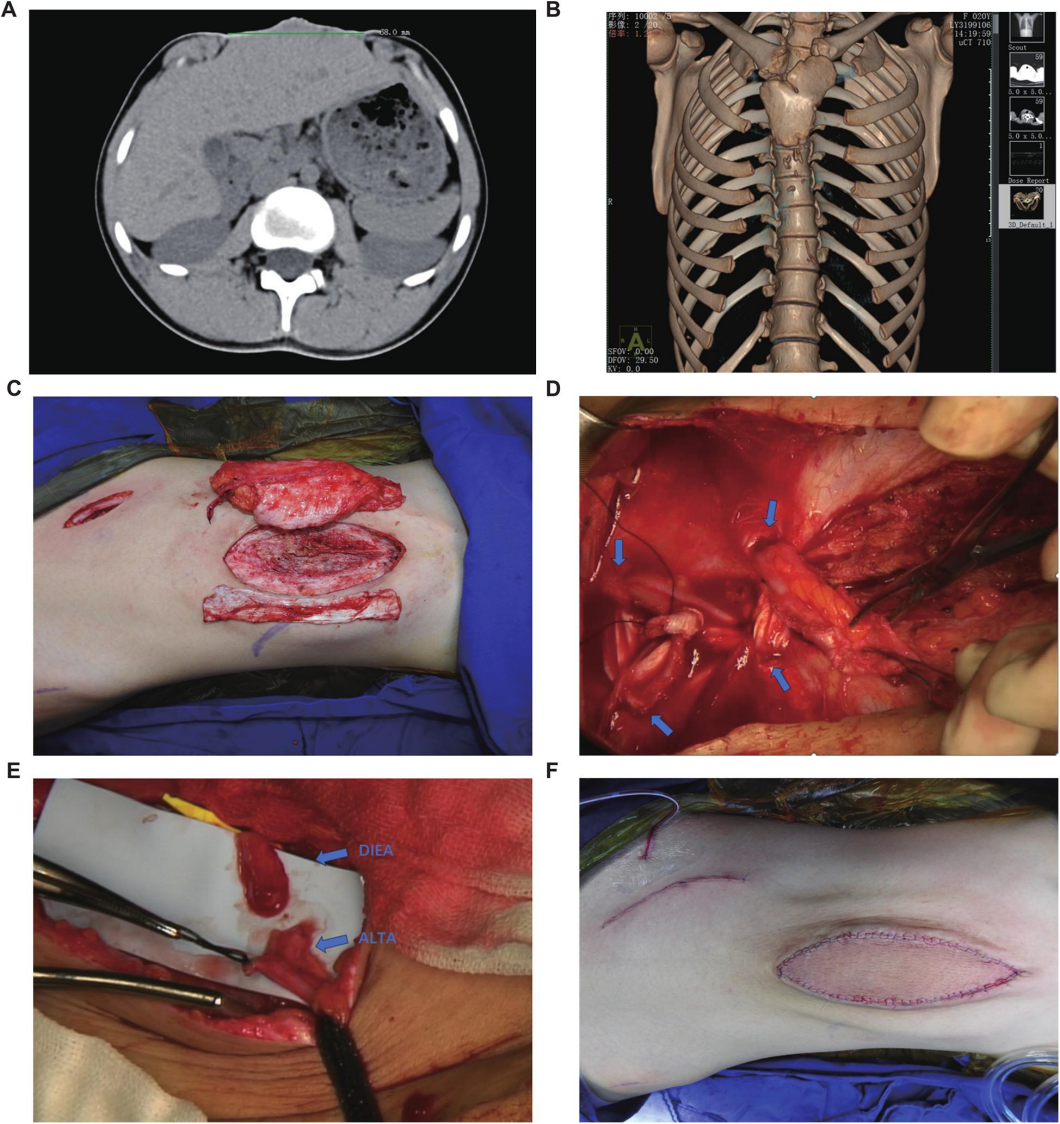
Preoperative examination and surgical procedure. (A) Abdominal CT suggests that the maximum diameter of the abdominal defect is 68 mm. (B) Chest CT scan (non-contrast) with three-dimensional volume rendering reveals asymmetric bilateral clavicles, sternal cleft with associated rib hypoplasia, and scoliosis. (C) The selected autologous free anterolateral thigh flap with fascia lata. (D) The inferior end of the rectus abdominis muscle was interwoven with the fascia lata. The blue arrow indicates the fenestration site in the rectus sheath. (E) The anastomosis site between the perforating branch of the deep inferior epigastric artery (DIEA) and the perforating branch of the anterolateral thigh artery (ALTAP). (F) Postoperative abdominal photograph.
